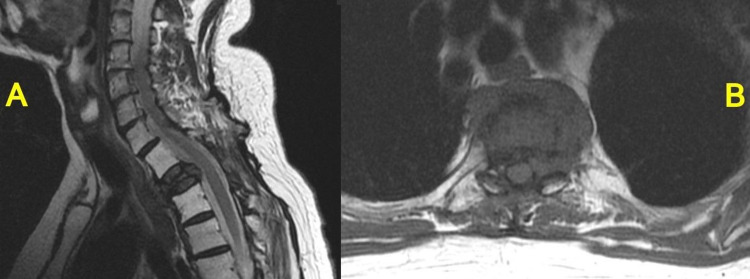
MRI five years two months after irradiation shows no recurrence. A:　T2-weighted image (sagittal)
